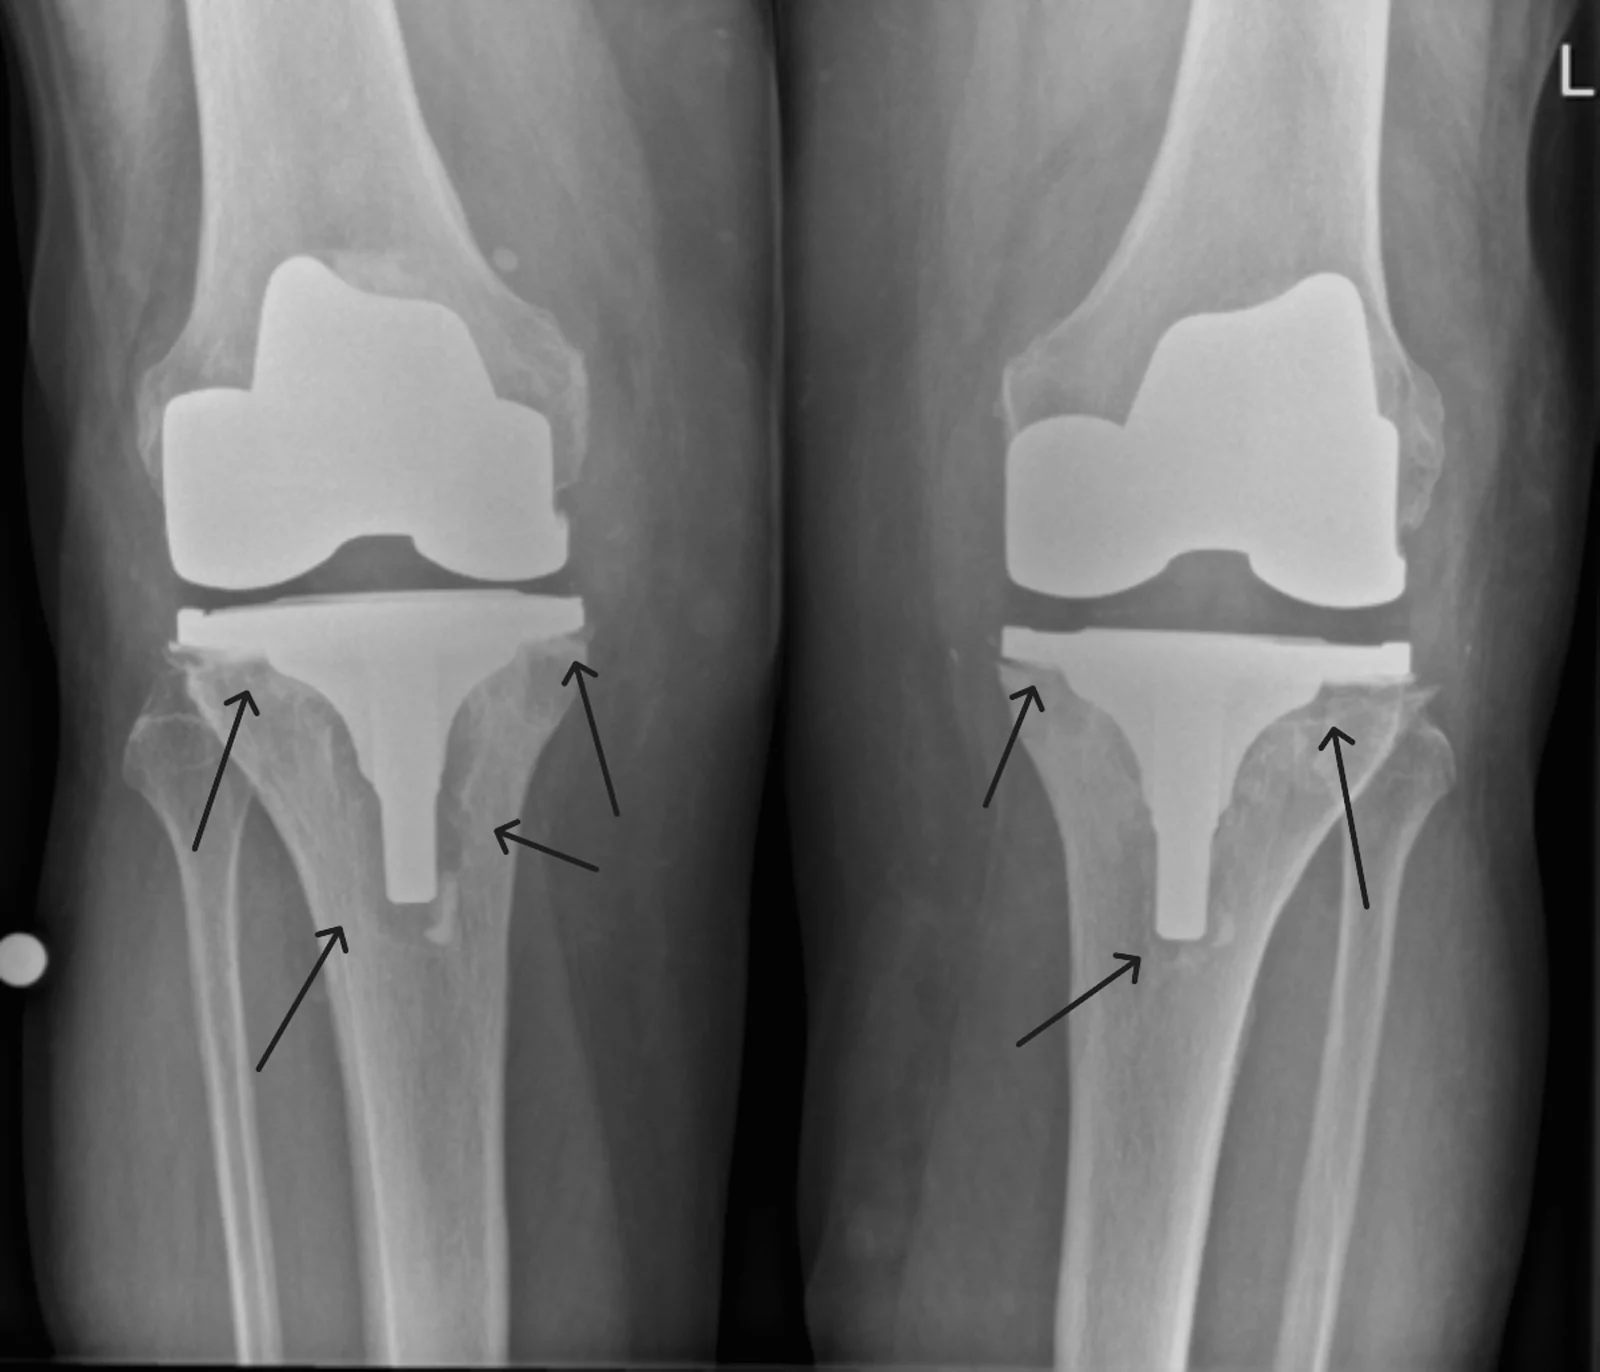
Anteroposterior radiograph of both knees showing minimal signs of osteolysis surrounding the TKAs five years after primary TKA. TKA, total knee arthroplasty
